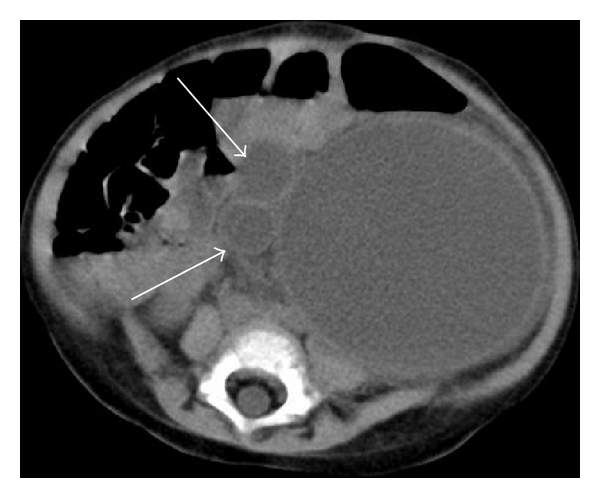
Computed tomographic scan of the abdomen showing a rounded appearance of the abdomen, markedly dilated and tortuous left ureter (arrows), and severe pelvicalyceal dilatation.
